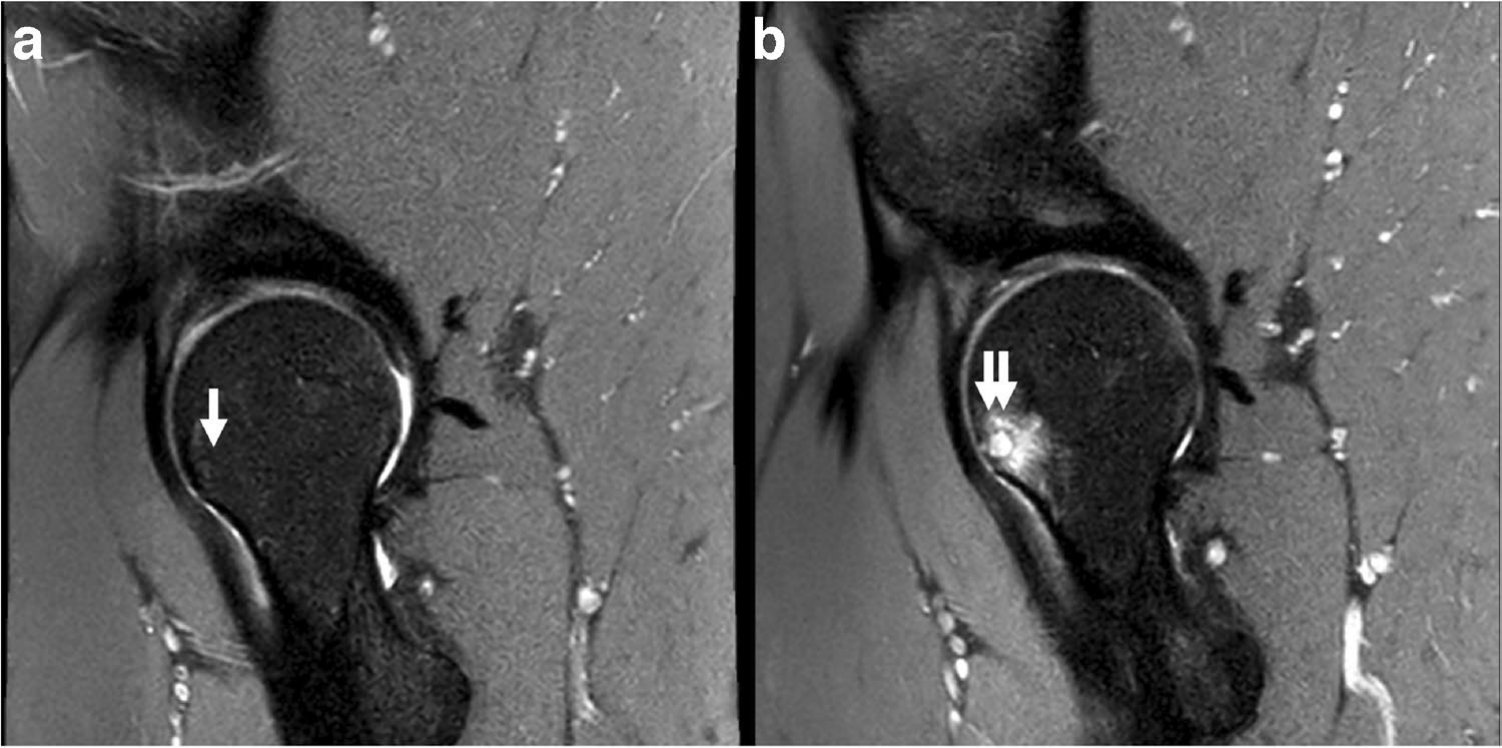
Incident subchondral cyst and BML. a Normal subchondral bone (white arrow) in the femoral anterior subregion. b Two-year follow-up MRI shows incident grade 2 subchondral cyst and grade 3 BML (double white arrow) in the femoral anterior subregion
